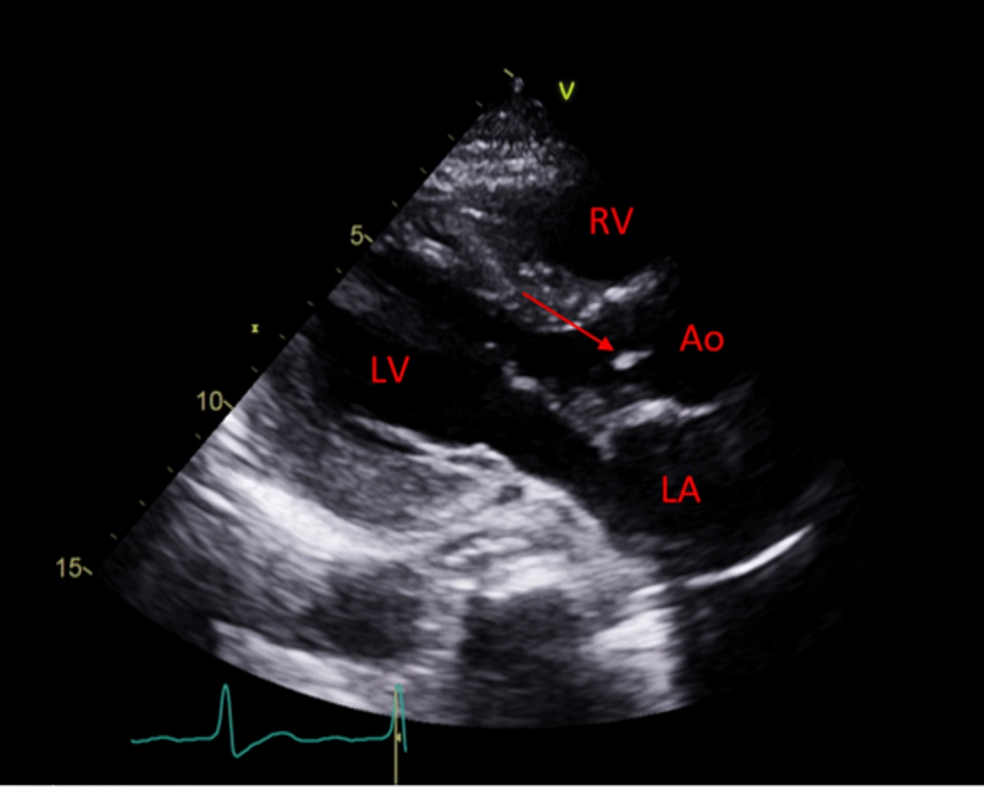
Transthoracic echocardiogram with parasternal long-axis view depicting an echogenic mobile structure tethered to the aortic valve.
